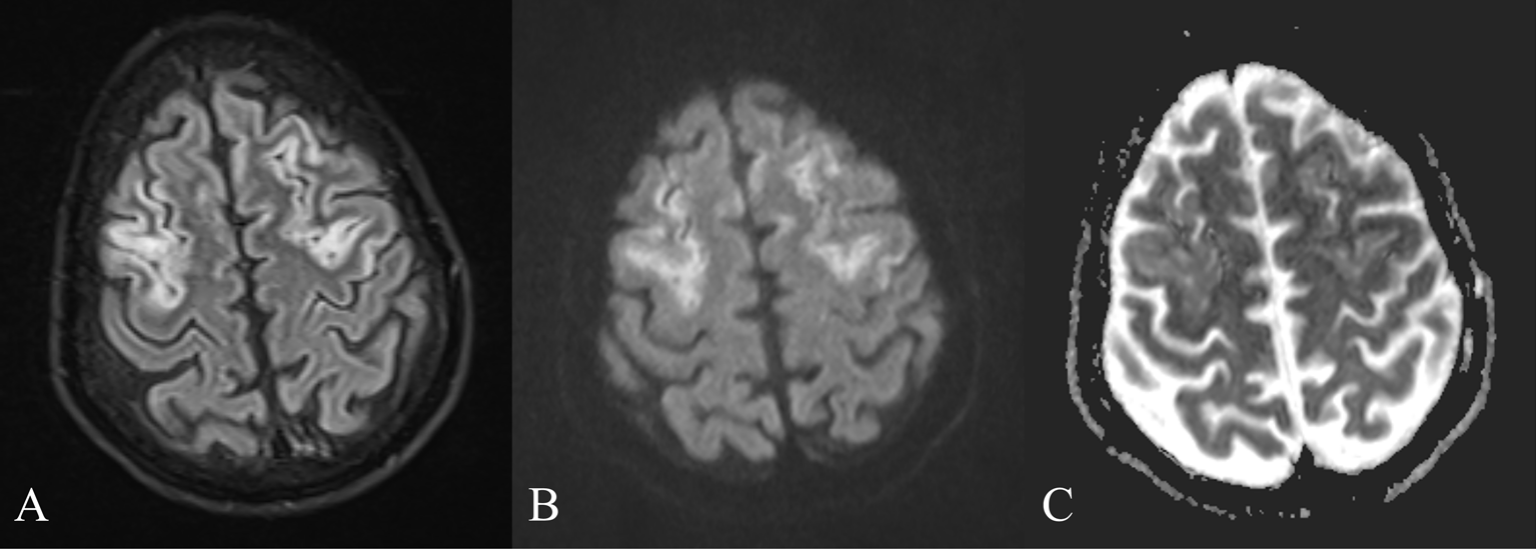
FLAIR, DWI and ADC. (A) FLAIR image showing increased signal in the frontal cortex, bilaterally. (B) Increased DWI signal is present in the same areas. (C) ADC map without a corresponding low signal, ruling out an ischemic stroke.
